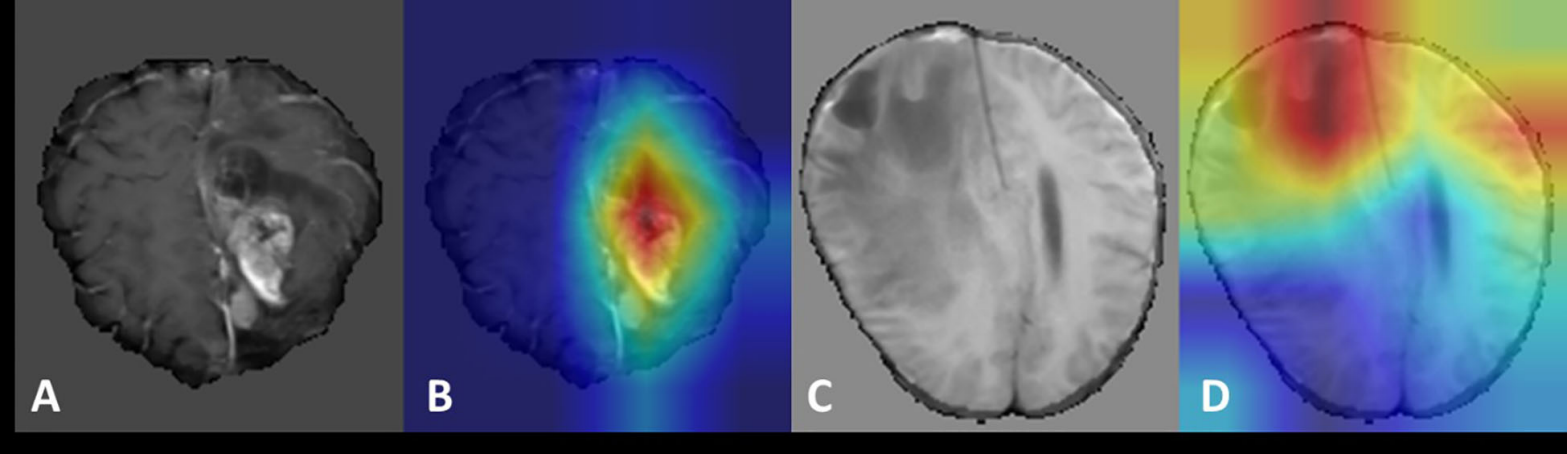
Visualization of recurrent glioma and radiation necrosis cases. (A, C) display the magnetic resonance images (MRI) after pre-processing such as skull removal used as model inputs for the recurrent glioma and radiation necrosis cases, respectively. (B, D) present the Grad-CAM visualizations for the recurrent glioma and radiation necrosis cases, respectively.
